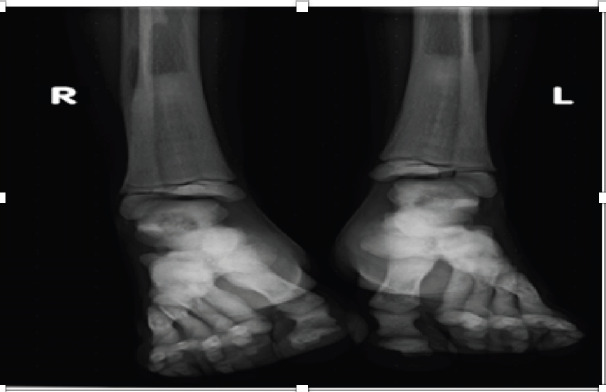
Bilateral frontal foot X-ray showing distal tibiofibular, tarsal, metatarsal, and phalangeal sclerosis
